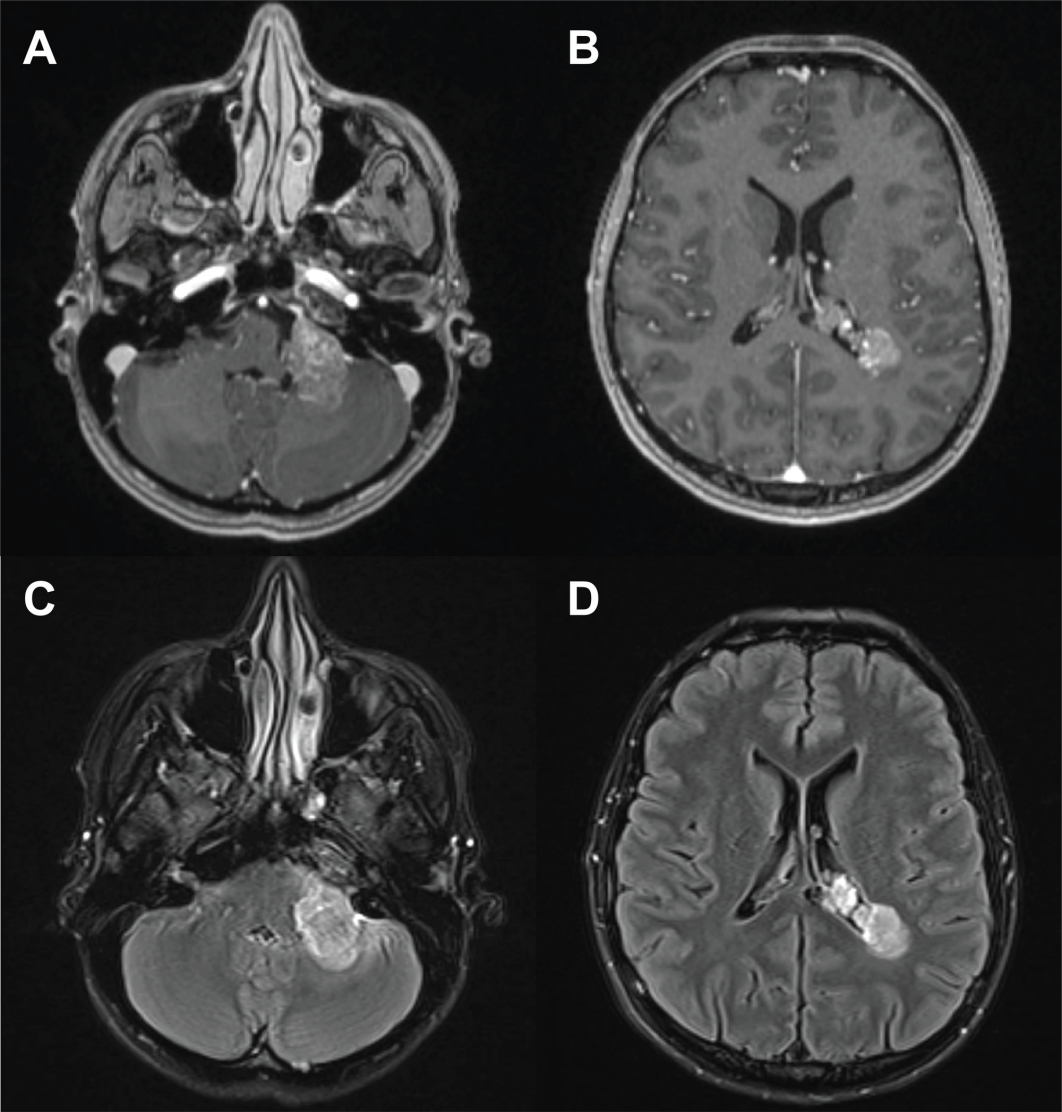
Preoperative brain MRI of the patient (A) Axial view of the left CPA mass located on T1. (B) Axial view of the lesion located in the atrium of the left ventricle on T1. (C) Axial view of the left CPA mass on T2-FLAIR. (D) Axial view of the left ventricle lesion on T2-FLAIR. MRI: magnetic resonance imaging; CPA: cerebellopontine angle; T2-FLAIR: T2-weighted-fluid-attenuated inversion recovery
